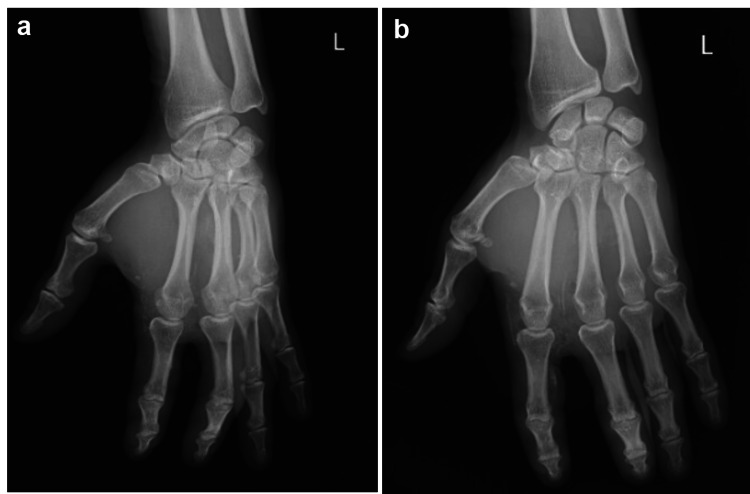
Case 3 (a, b) Postoperative X-ray of the left hand showing no residual material.
